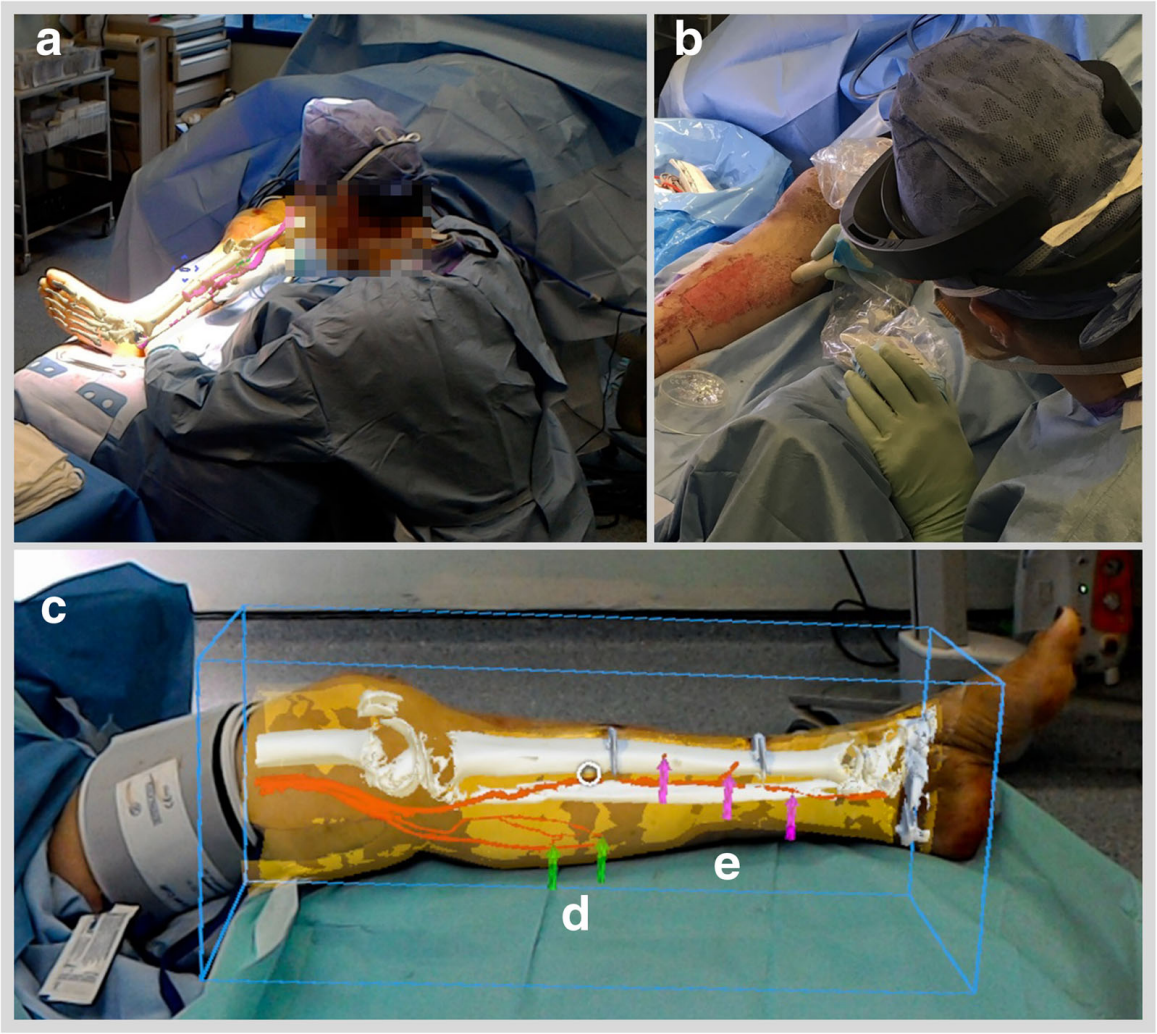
a Case 3 AR overlay of models as viewed from remote HoloLens; (b) confirmation of perforator location with audible Doppler ultrasonography. c Case 6 overlay with bounding box; *arrows* highlighting position of (d) medial sural and (e) posterior tibial perforators
